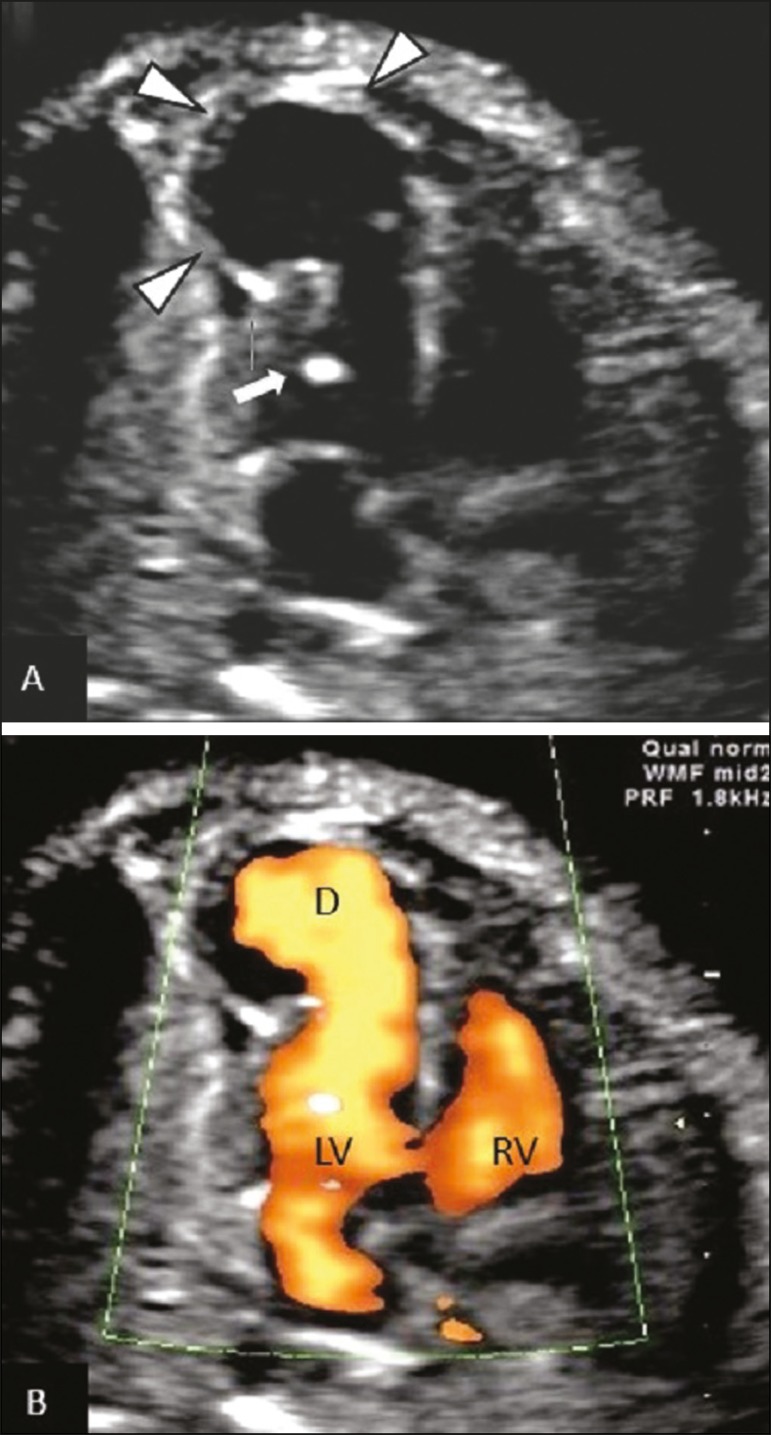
Four-chamber ultrasound view of fetal heart. A: Diverticulum
